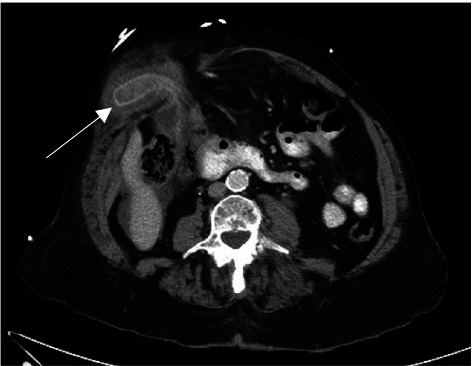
Computed tomography scan of gallbladder fundus herniation into parastomal hernia (arrow) with thickened fundal wall and pericholecystic fluid in axial plane.
